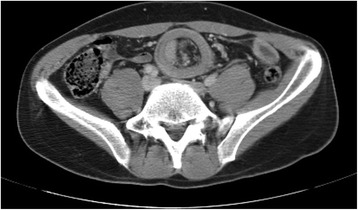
Typical image of "target sign" in the CT scan
